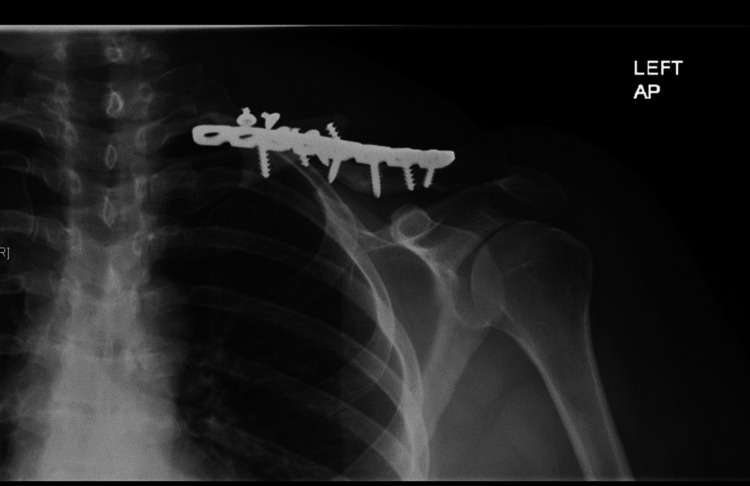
Pre-operative anteroposterior x-ray of the left clavicle showing hardware failure and nonunion.
